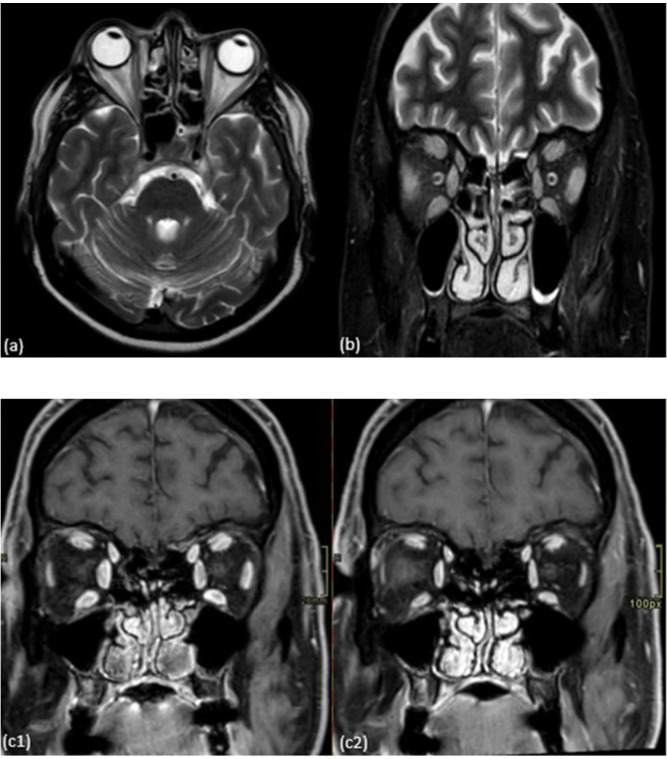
(a) MRI of the brain. (b) Axial T2W images of the brain demonstrates bilateral proptosis, right more than left. (c) Coronal STIR images through the orbits show increased signal intensity within the bilateral intraocular muscles suggestive of oedema. Coronal post contrast SPIR images of the orbits showing increased thickness of the intraocular muscles in October 2022 (c1) compared to images from April 2017 (c2).
